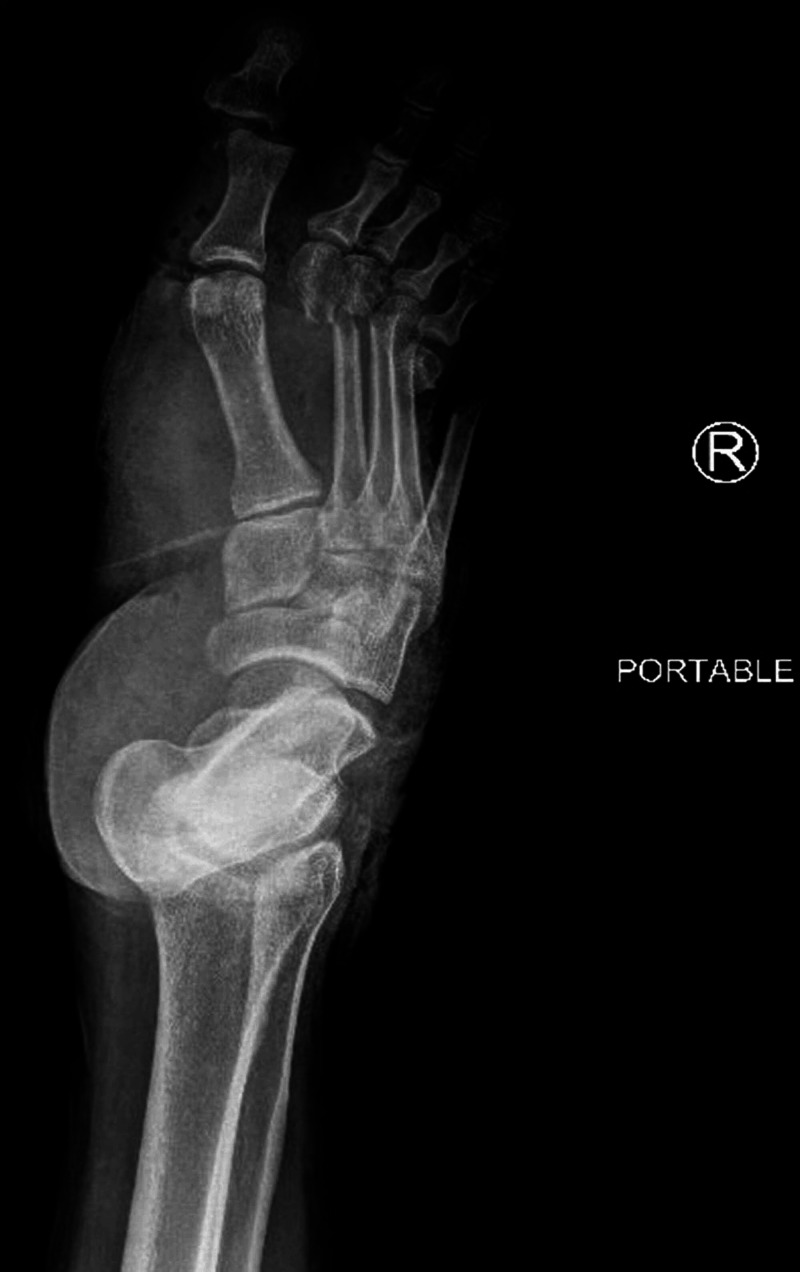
Plain film x-ray of the patient's crushed foot obtained in the trauma bay Plain film x-ray of the patient's right lower extremity. Note the displaced fractures of metatarsals 2-4, displaced right medial malleolar, and tibial metaphysis fractures.
